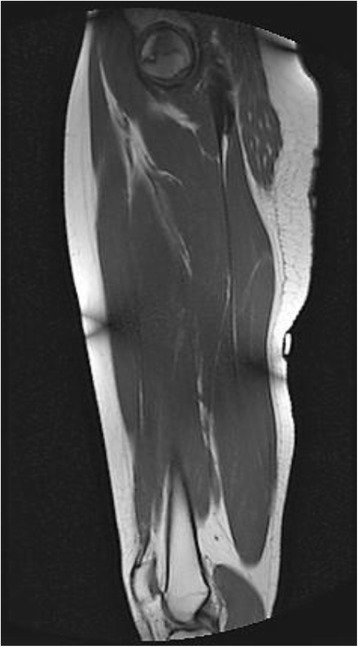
This T1-weighted image shows a serpiginous band of hypodensity that represents the separation point between necrotic bone and bone that is undergoing repair. This is pathognomonic of AVN
